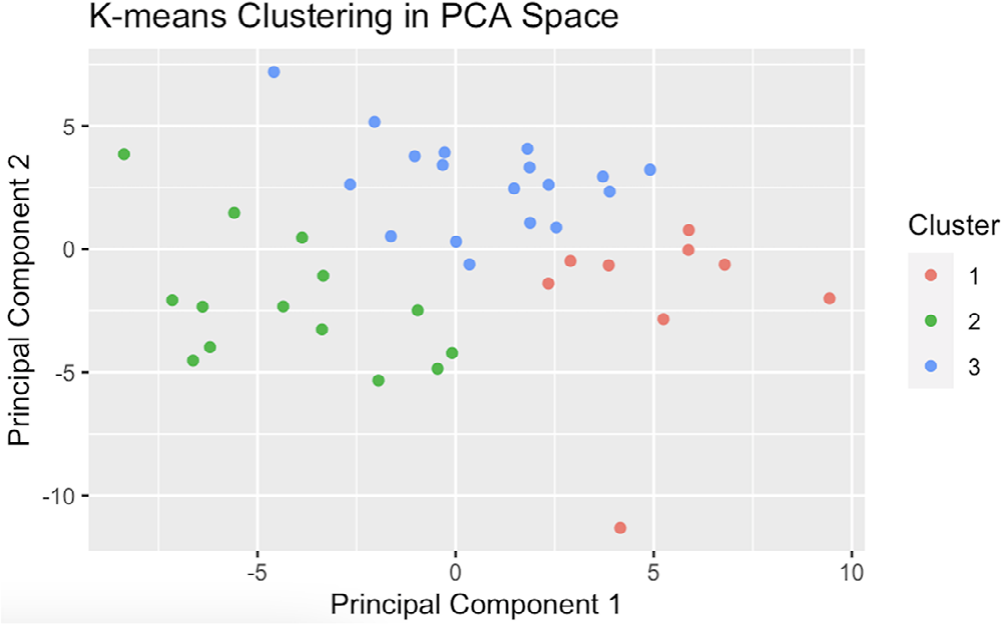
# Is FDG‐PET metabolism associated with cognitive performance in long‐Covid patients?
